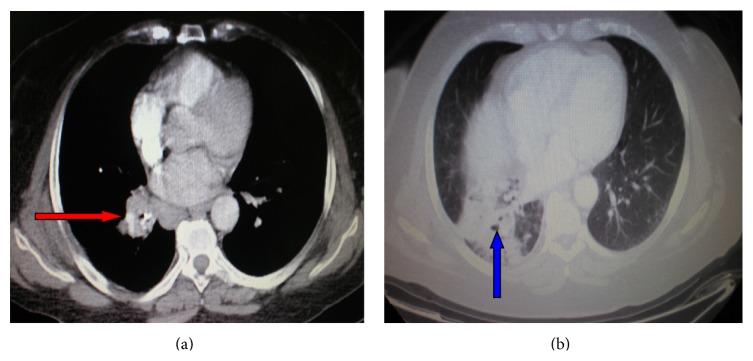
(a and b) A contrast-enhanced computed tomography of the thorax revealing a right hilar mass (red arrow) compressing the bronchus intermedius with accompanying dense airspace opacification of right lower lobe (blue arrow) and atelectasis.
